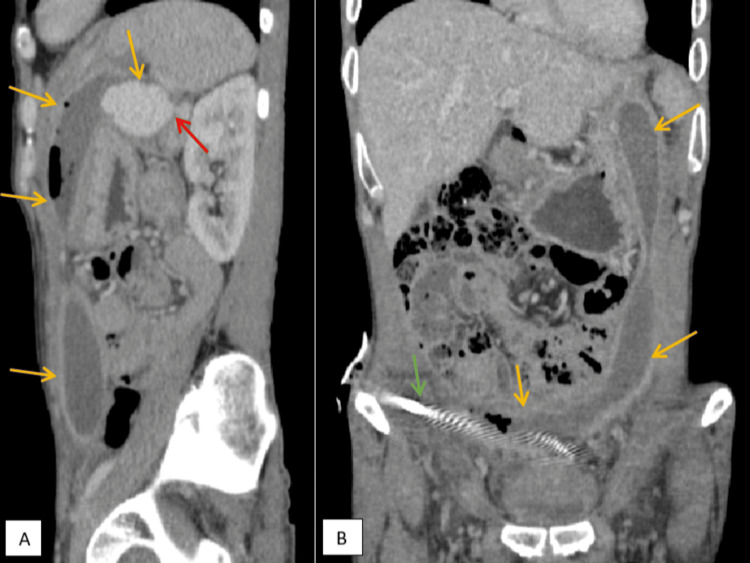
Sagittal (A) and coronal (B) reformatted images from the venous phase of a contrast-enhanced CT abdomen in a 62-year-old male show interconnected, walled-off fluid collections along the left paracolic gutter and pelvis (yellow arrows). The pigtail is seen within the pelvic collection (green arrows). A well-defined lesion is seen within the collection arising from the tail of the pancreas, whose contrast enhancement mirrors that of the splenic vein (red arrow). The lesion is seen arising from the splenic vein, protruding into the pancreatic pseudocyst.
